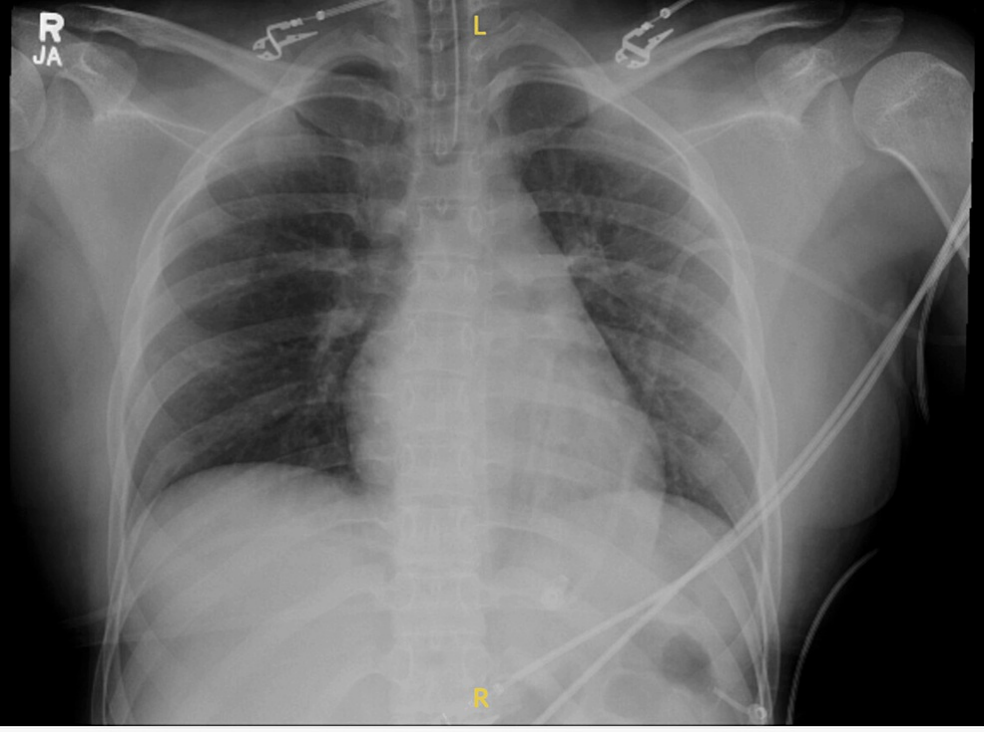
Chest radiography (Chest X-ray) of Patient X.
